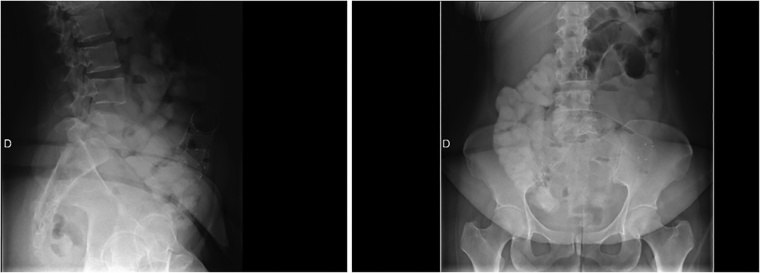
Radiographs of the migrated self-expandable gastric stent.
